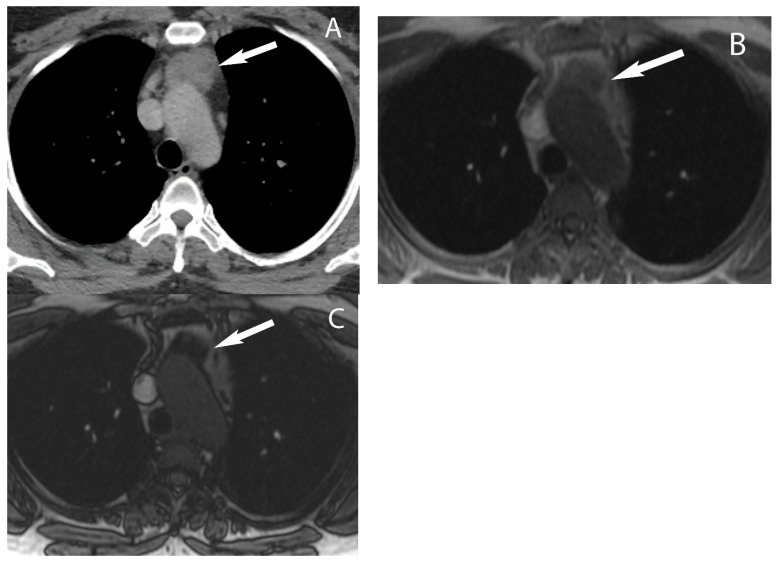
Thymic hyperplasia. CT (A) shows a rounded soft tissue mass in the prevascular mediastinum (arrow) in a woman with breast cancer. Differential diagnosis includes thymic epithelial neoplasm or metastatic disease. MRI chest in-phase image (B) shows the mass is isointense to muscle (arrow) and shows drop in signal on opposed-phase image (C) consistent with thymic hyperplasia.
